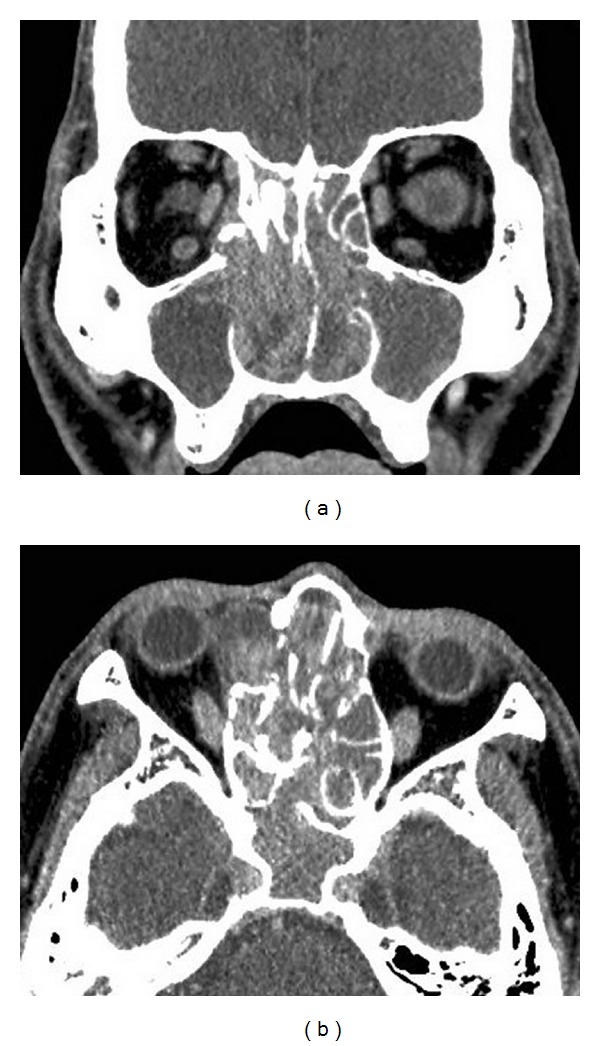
Enhanced computed tomography (CT) image (a, b). A homogeneously enhancing mass that filled the bilateral nasal cavities and the maxillary and ethmoid sinuses. Bone destruction of the right orbita was observed. Osteogenesis was observed in the right ethmoid sinus.
